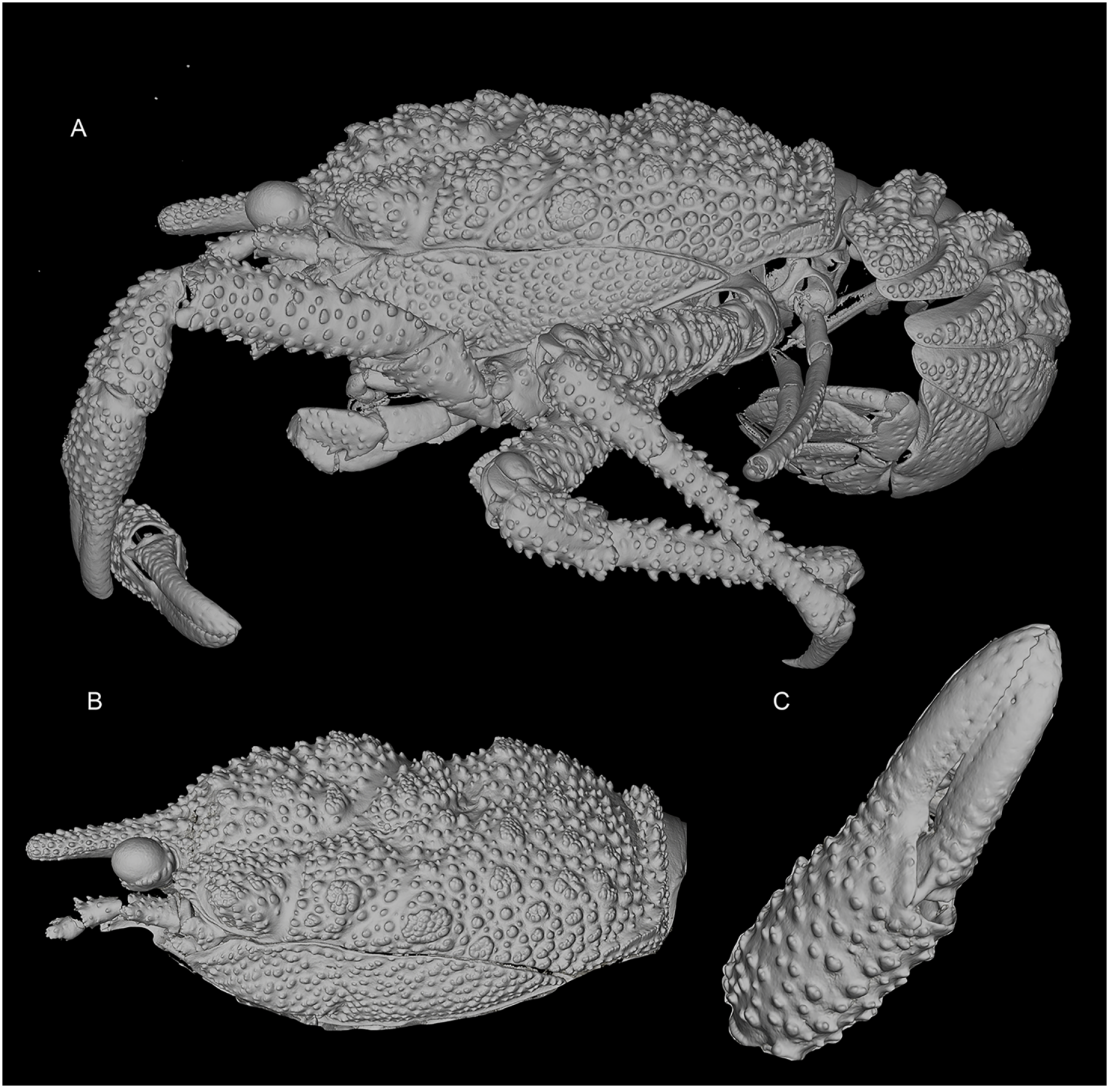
General habitus and details of the new species. 3D-renderings of micro-computed tomography x-ray images. *Munidopsis hawaii*
